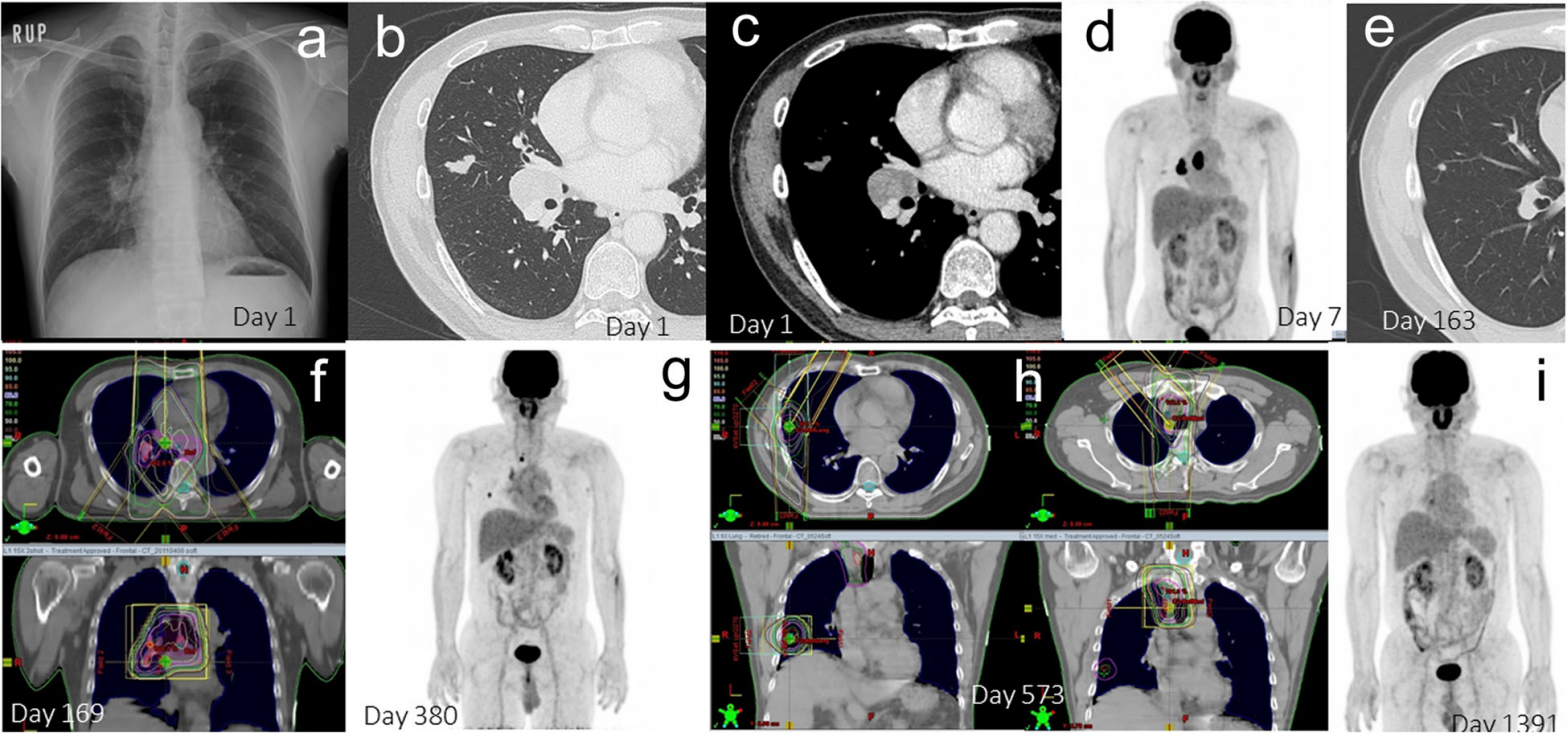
A representative complete remission (CR) patient with oligometastases in stage III. A CR patient with stage III (a-i), in whom successful control of primary and oligometastatic lesions was obtained, is shown. A male in his 50s consulted our hospital due to an abnormal shadow recognized on a chest X-ray (a). The computed tomography (CT) revealed the primary lesion to be in the right middle lobe (b), as well as swelling of a right hilar (c) and a mediastinal lymph node. Positron emission tomography (PET) / CT (d) and head magnetic resonance imaging (MRI) demonstrated the patient to have stage IIIA (cT1bN2M0) adenocarcinoma. The primary lesion showed scar formation after chemotherapy (e). However, PET/CT showed viable cells in the right hilar lymph node. Three-dimensional conformal radiation therapy was planned for the right hilar and mediastinal lymph nodes (f). On the day 380 since initial treatment, PET/CT revealed recurrence of the primary lesion and a new mediastinal lymph node metastasis (g). Three-dimensional conformal radiation therapy was administered for these lesions (h). The PET/CT (i) and the head MRI demonstrated CR
